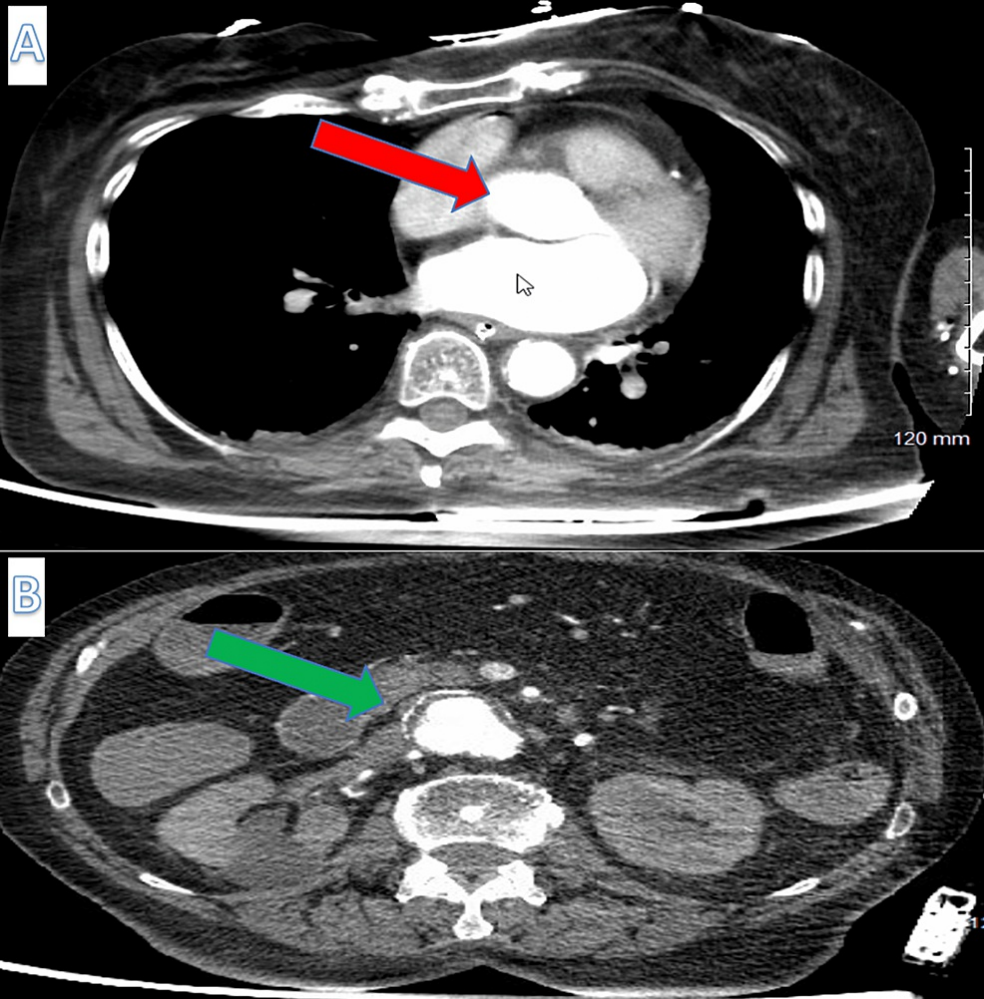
Computed tomography angiogram (CTA) chest and CTA abdomen and pelvis of the patient. The images show (A) CTA chest showing an unruptured 1.7 x 0.7 x 1.3 cm saccular aneurysm of the posterior wall of the ascending aorta (red arrow) and (B) CTA abdomen and pelvis showing diffuse aneurysmal dilation (green arrow) of infrarenal abdominal aorta measuring 4.4 x 4.3 cm with no leak or rupture along with a short segment of aortic dissection flap involving 4 cm long segment of aorta.
